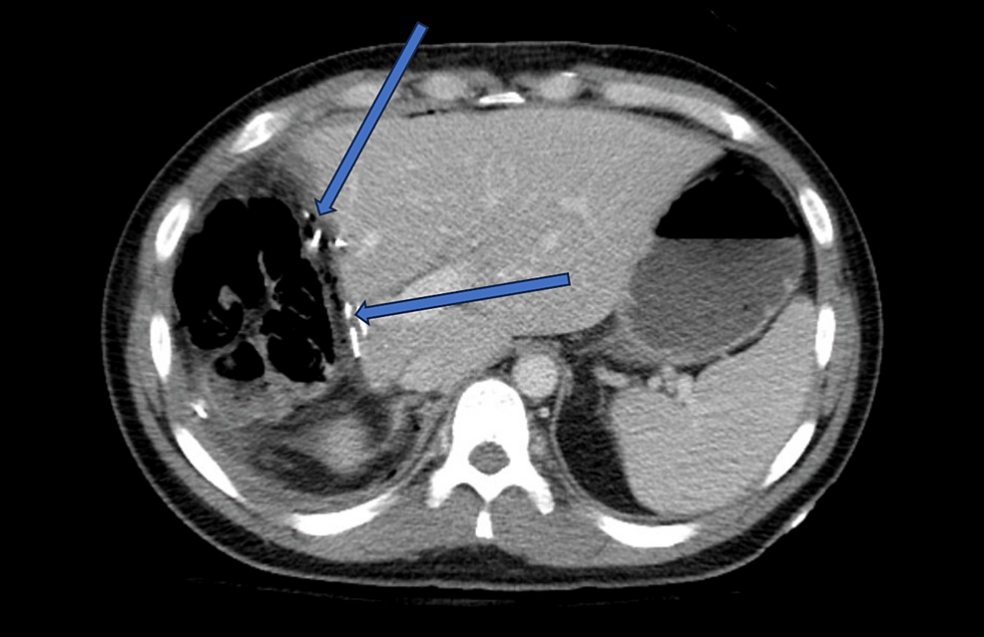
CT scan of abdomen and pelvis With IV Contrast Status post right hepatectomy for FLHCC
